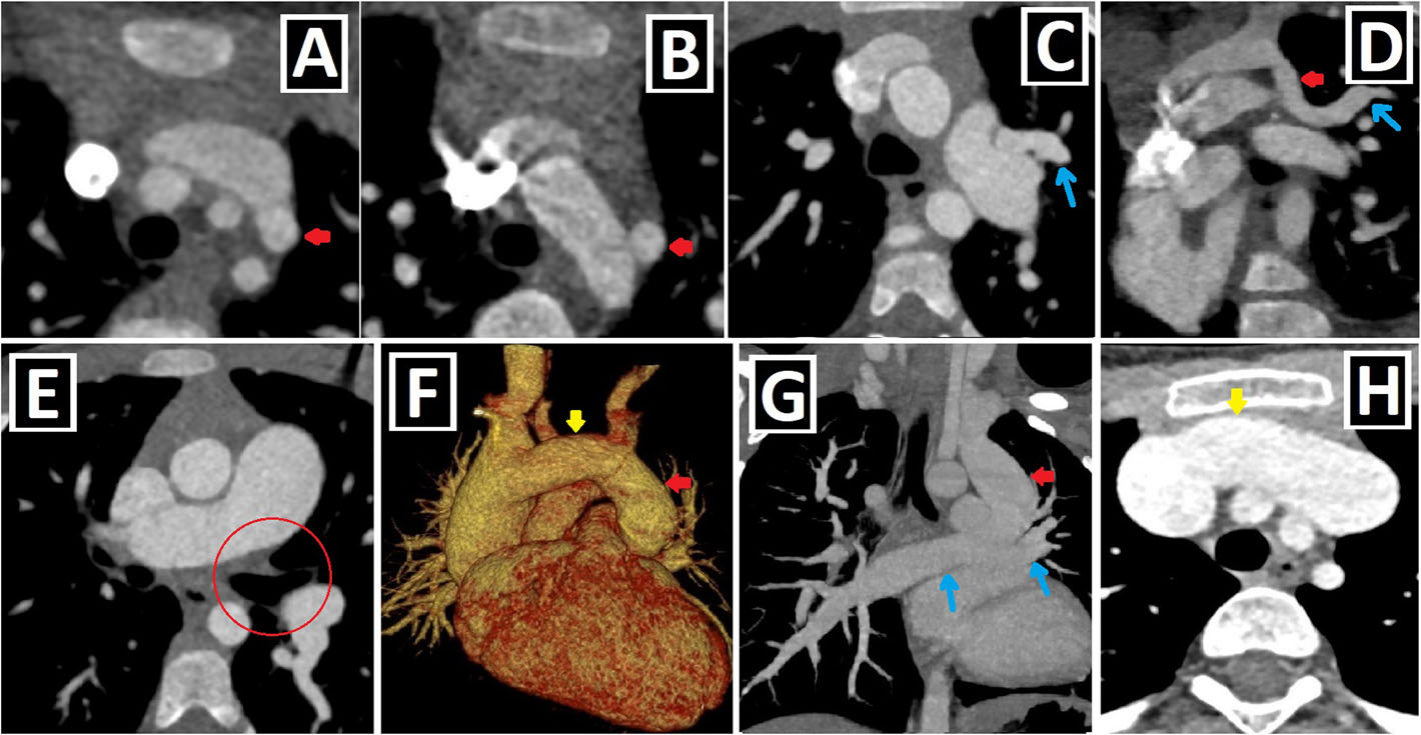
Vertical vein. a–e Axial (a, b, c, e) and coronal-oblique reformated (d) CT images depict abnormal drainage of the left upper pulmonary vein (blue arrows) into the left brachiocephalic vein via the VV (red arrows). In the presence of VV with PAPVD (left-upper), the absence of vascular structure anterior to the left main bronchus (red circle) is an important clue for differential diagnosis (e). f–h 3D VRT (f), coronal-oblique maximum intensity projection (g), and axial (h) CT images depict the drainage of both right and left pulmonary veins (blue arrows) into the VV (red arrows), in a patient with supracardiac type TAPVD. The VV transports the whole pulmonary venous blood to the SVC through a large-caliber bridging vein (yellow arrows)
